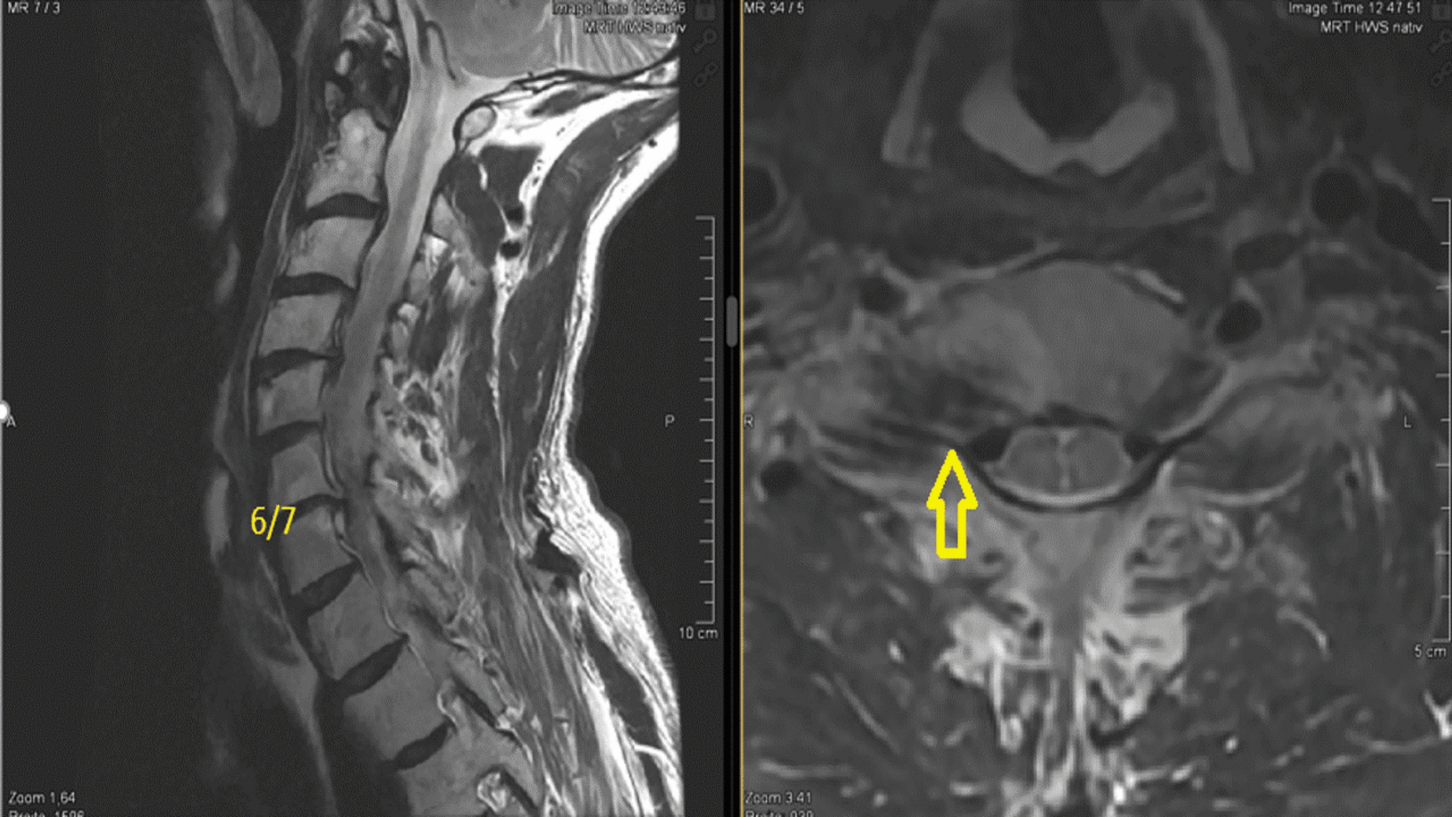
Preoperative T2-weighted MRI (sagittal and axial views) showing right-sided neural foraminal stenosis at the C6-C7 level (yellow arrow). MRI, magnetic resonance imaging
